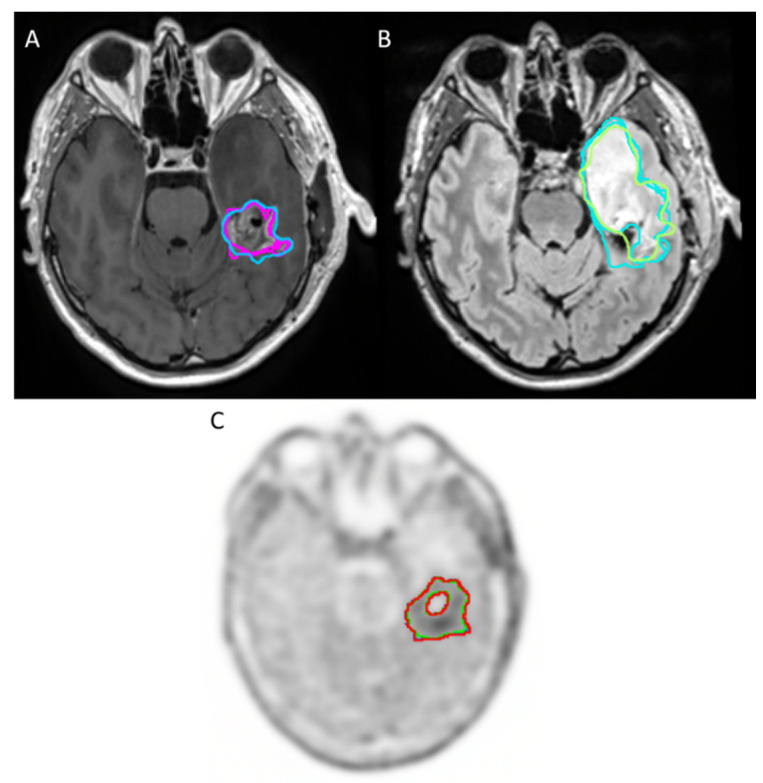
Example of sequences with good agreement for tumor volume delineation in high-grade glioma between readers: (A) CE T1-weighted imaging (T1-GD) (B) T2-weighted fluid-attenuated inversion recovery (T2-FLAIR), which appears in hypersignal. (C) 18F-FET PET/CT.
